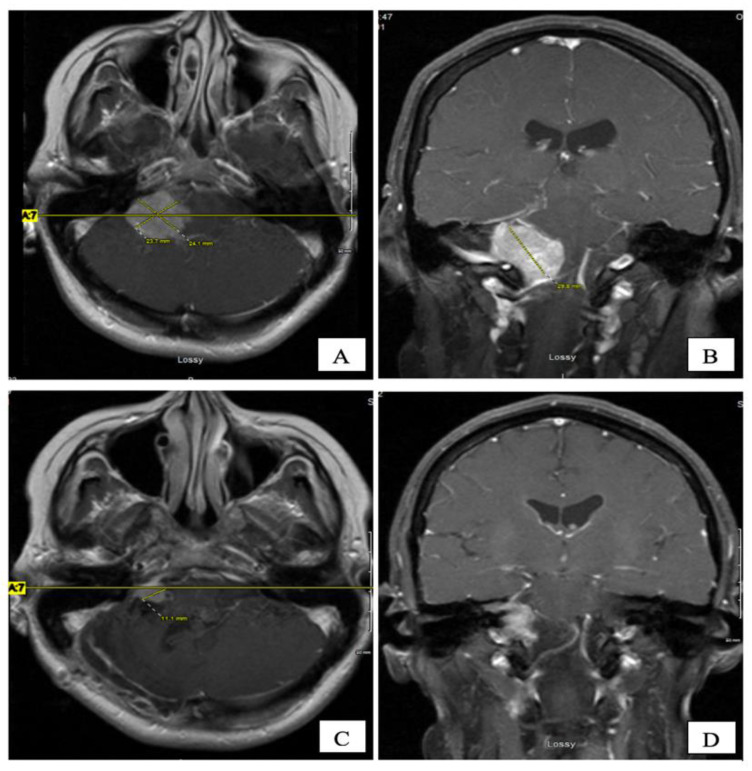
Pre-operative ((A) axial, and (B) coronal) and 2 months post-operative ((C) axial, and (D) coronal) post-gadolinium T1-W MRI sequences. The CPA tumour measured 24 × 24 × 30 mm and 11 × 9 mm, respectively.
